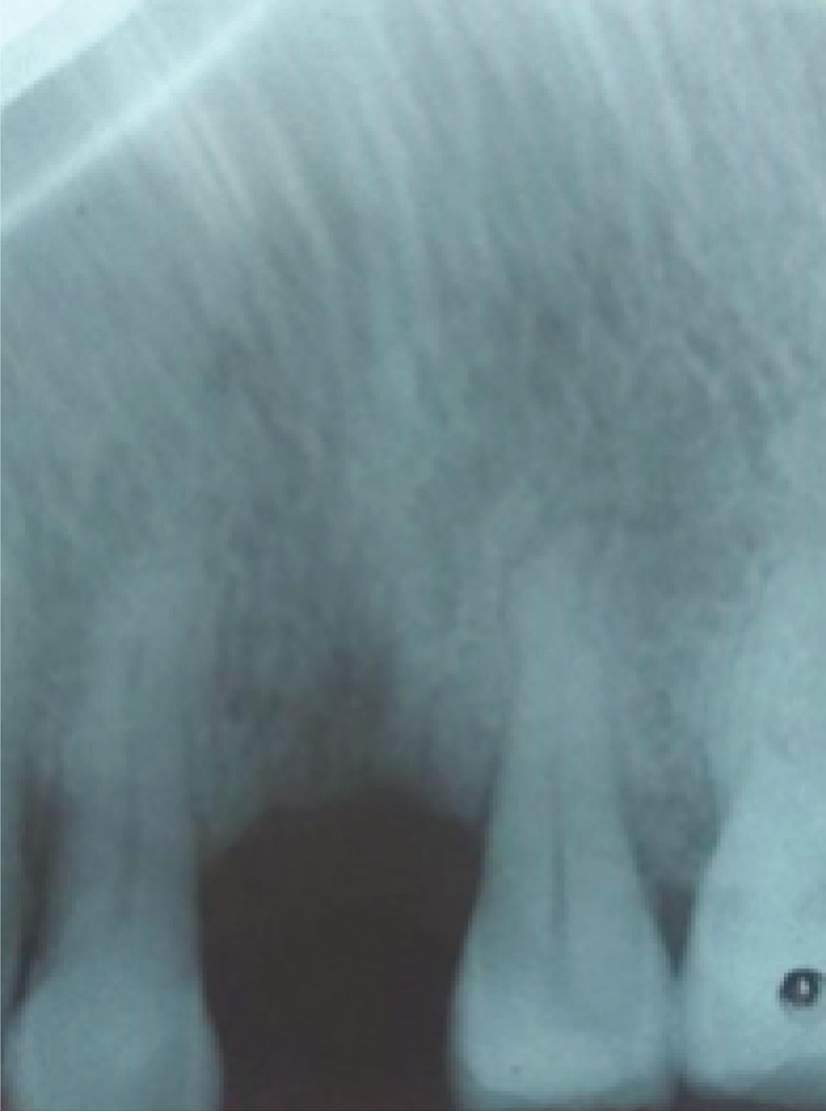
Initial X-ray showing bone loss levels in the right central incisor.
